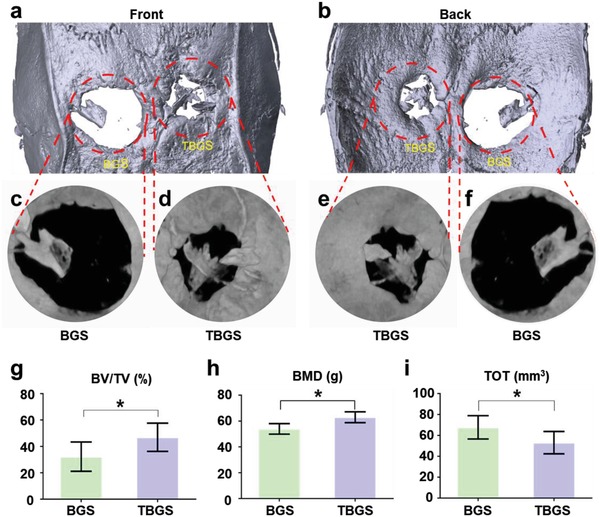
In vivo osteogenesis performance of BGS and TBGS. a,b) 3D reconstruction of circular defects at 24 weeks after scaffolds implantation. c–f) Micro‐CT images of cranial defect areas with a diameter of 5 mm at 24 weeks postoperation. g) Value of BV/TV in newborn osseous tissue (*n* = 6). h) Value of BMD in newborn osseous tissue (*n* = 6). i) Value of TOT in newborn osseous tissue (*n* = 6). p* < 0.05, *p* < 0.01, p* < 0.001.
